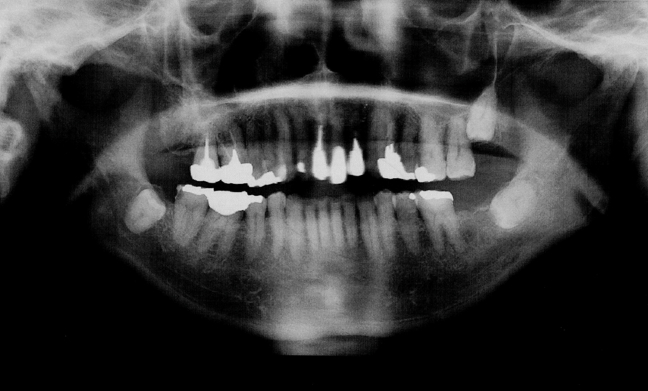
Panoramic x-ray Oral status of teeth with caries-like opacities and dental history of root canal treatment, prosthetic treatment, and tooth extraction.
